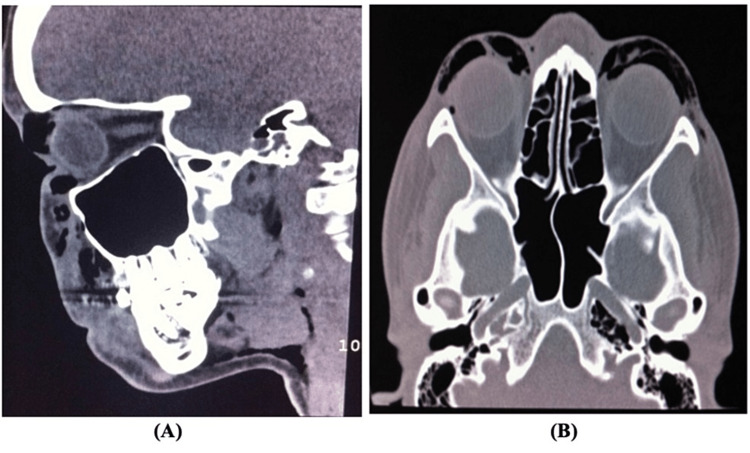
CT brain image showing per-orbital emphysema without crepitation after closed-structured rhinoplasty. (A) CT (sagittal) showing emphysema in the per-orbital area. (B) CT (axial) showing emphysema in the per-orbital area. Image credit: Charles-de-Sa et al., 2014 [29] with permission.
